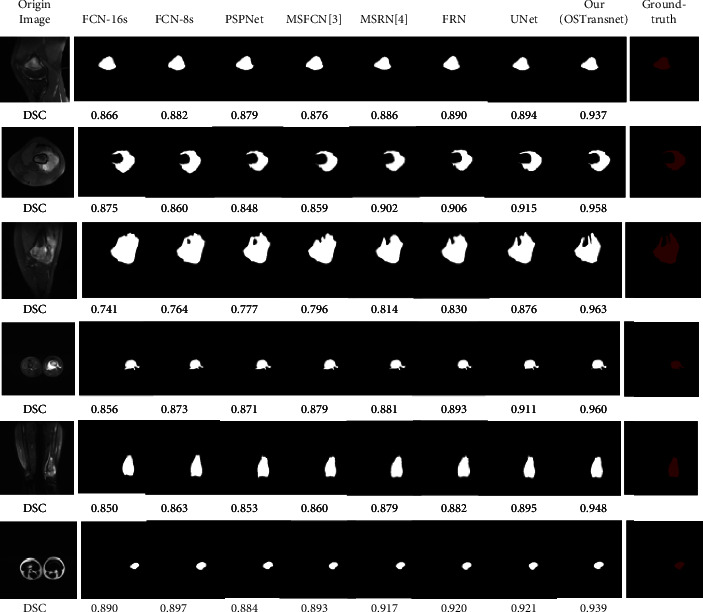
Comparison of the effect of each model on MRI image segmentation of osteosarcoma.
